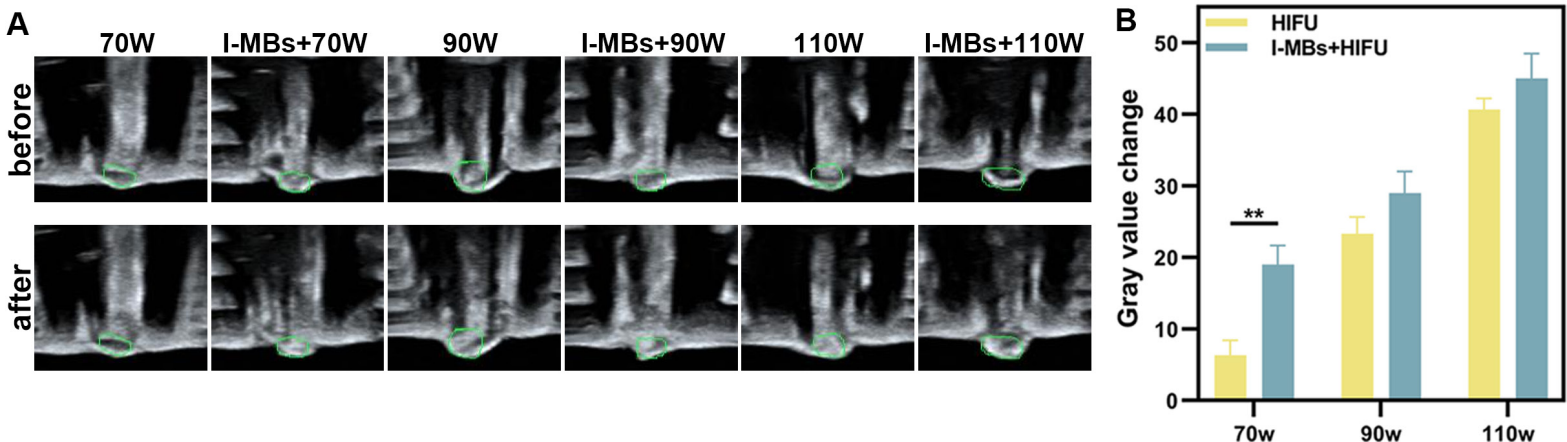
Optimization of Acoustic Power (A) Real‐time ultrasound images before and after HIFU irradiation on tumors at 70, 90, and 110 W for 2 s. (B) The quantitative analyses of A (*n* = 3, *p* < 0.01).
